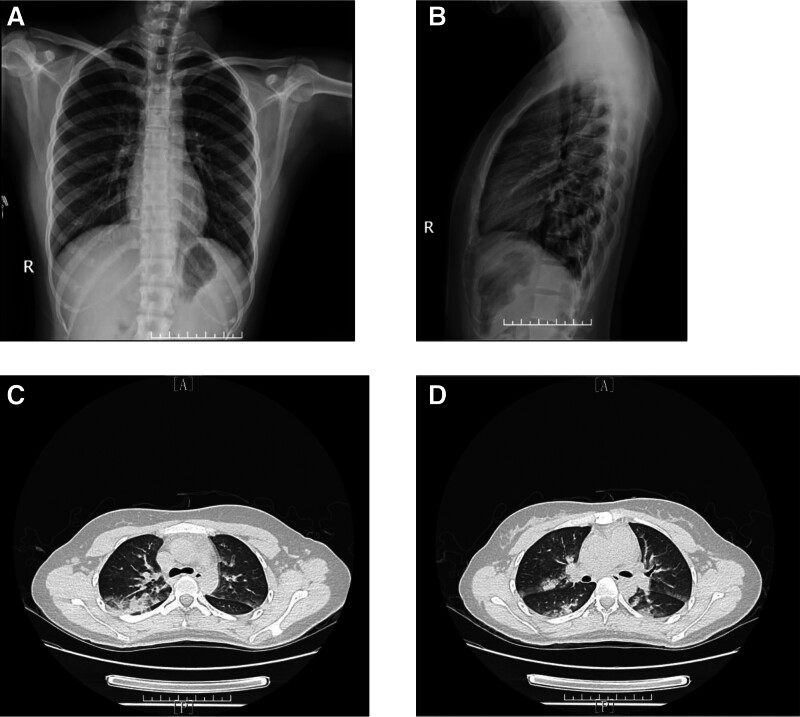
(A and B) Patient preoperative chest radiograph, no significant abnormality; (C and D) patient chest CT after NPPE, massive patchy hyperdense shadow in both lungs. NPPE = negative pressure pulmonary edema.
